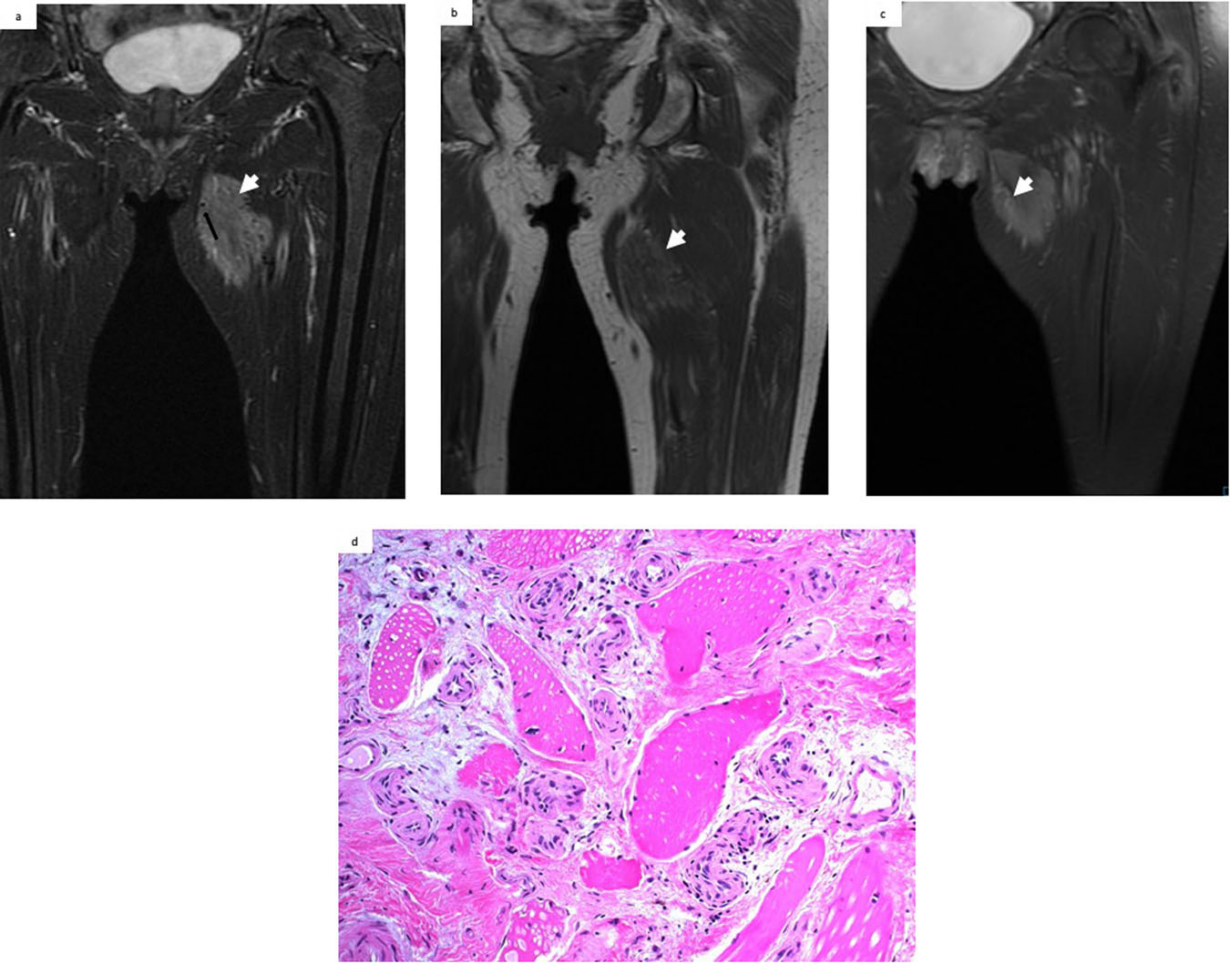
MRI in a 53-year-old man with a painful mass on his left thigh. a Heterogeneous hyperintensity appearance of the intramuscular mass (white arrow) on T2WI with fat suppression with flow voids (black arrow). b Hypointensity appearance of the intramuscular mass on T1WI (white arrow). c Moderate enhancement (white arrow) on delayed contrast-enhanced T1WI with fat suppression. d A non-involuting congenital hemangioma (NICH) confirmed by pathological analysis
